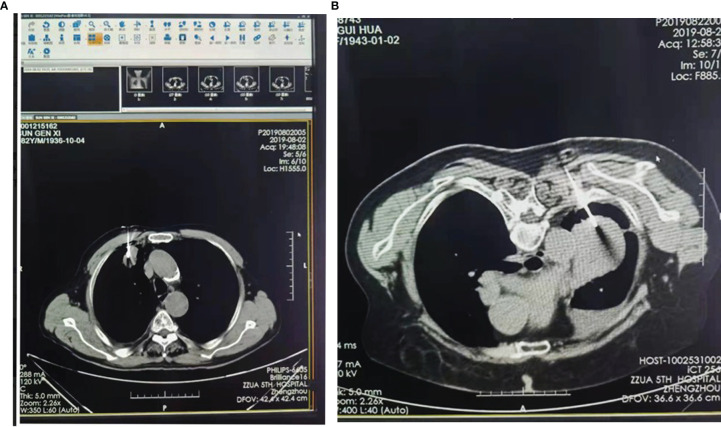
Two case of CT-guided lung puncture. (A) Lesion diameter of 2-5 cm; (B) Lesion diameter >5cmssssss.
